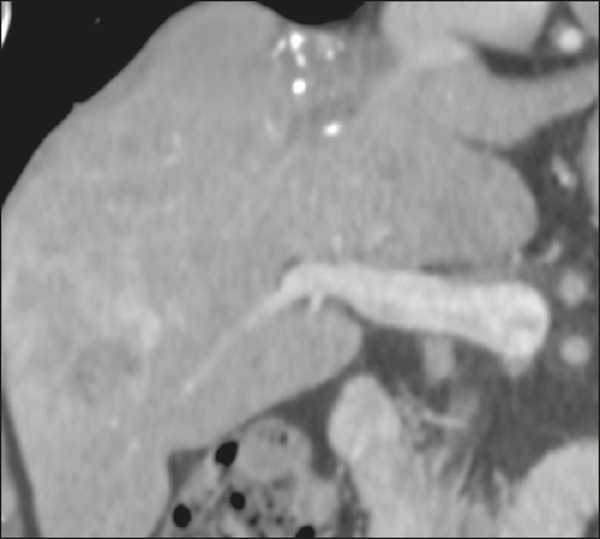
T2-weighted MRI sequence showing liver metastases with a cystic component exhibiting a fluid-fluid level.
